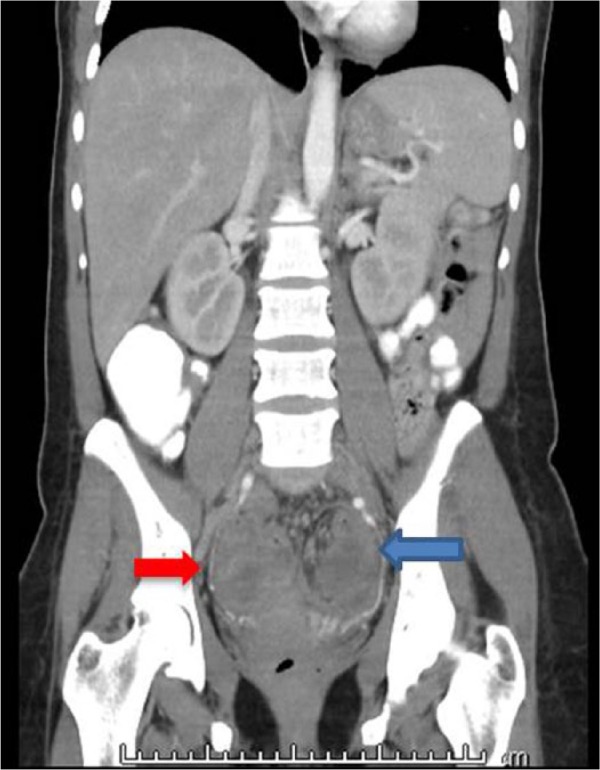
CT of the abdomen with contrast, coronal view.
